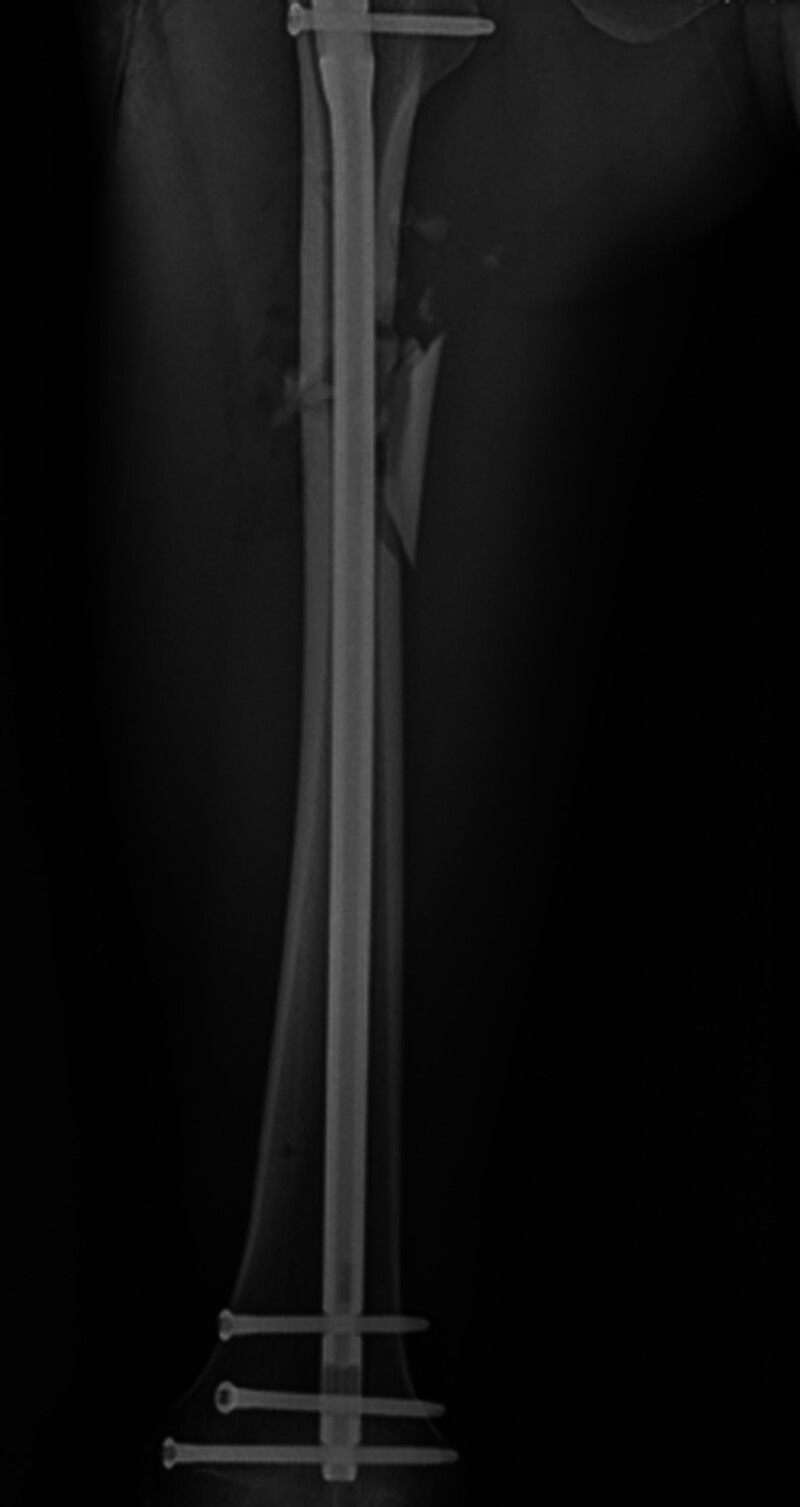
Postoperative x-ray showed satisfactory reduction.
